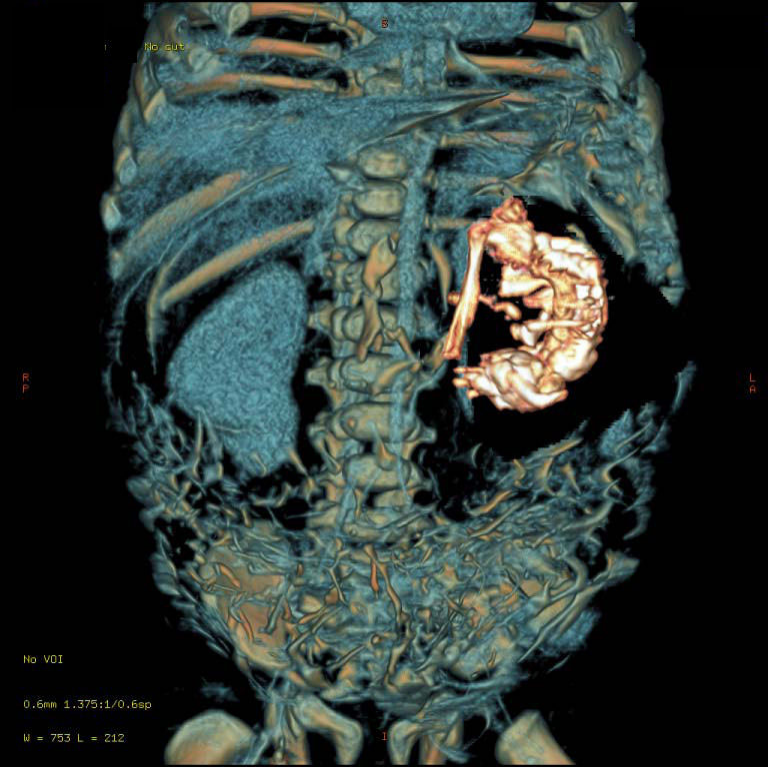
A 64-slice computed tomography scan of the bony outline of the fetus in fetu.
